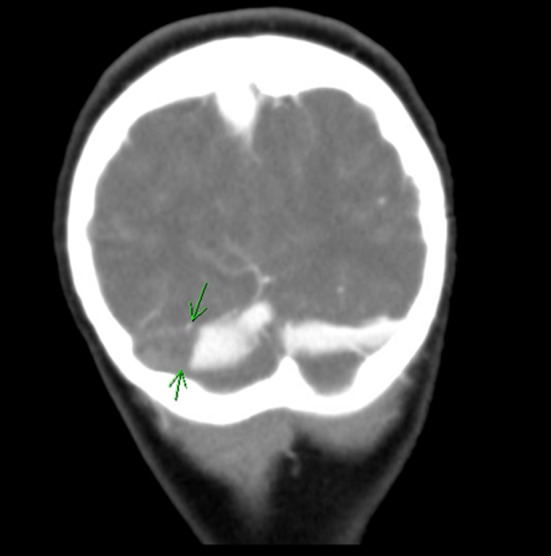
Head CT with contrast arrows indicate the thrombosis within the right transverse sinus; minimal contrast is seen past the thrombosis indicating near‐complete obstruction of the vessel
